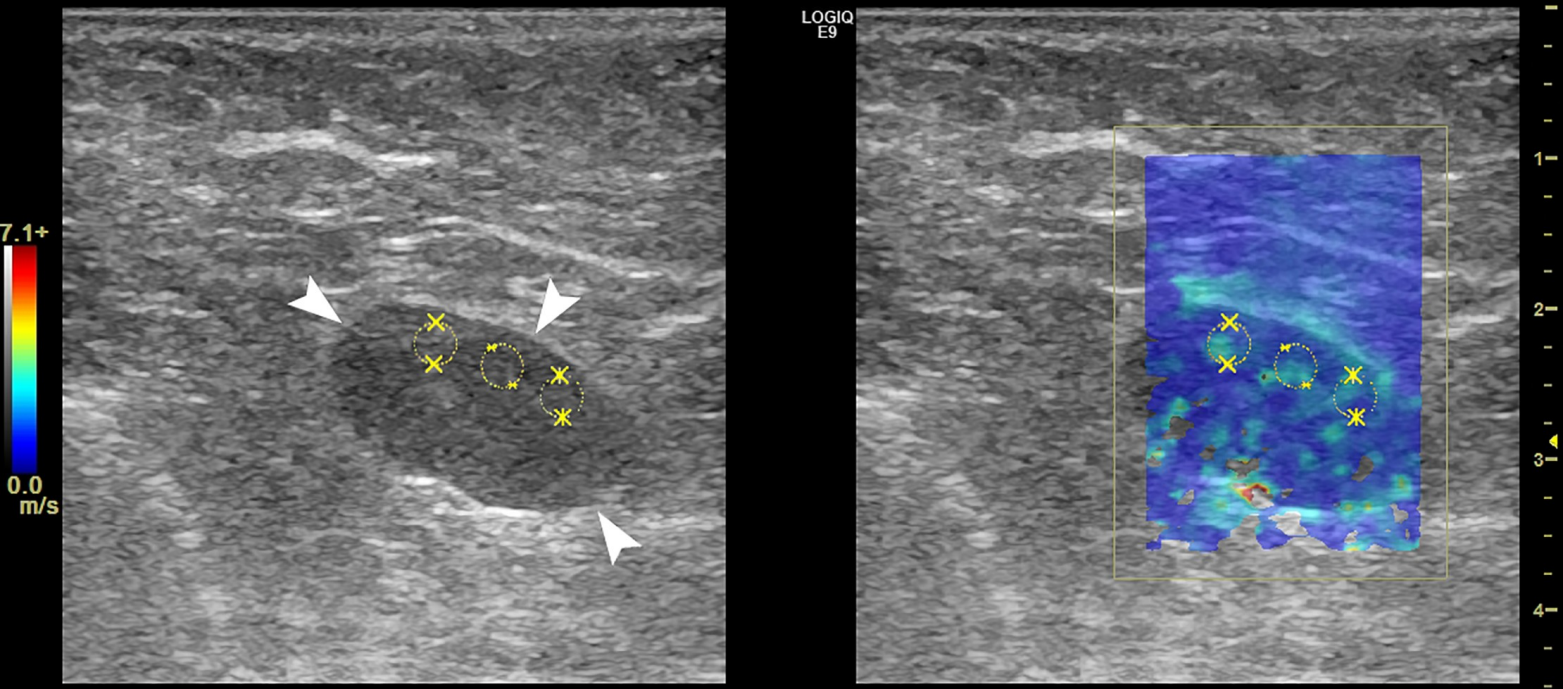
Left axillary B-mode (left) and shear wave speed map (right) images in a 60-year-old woman with a left breast mass and left axillary adenopathy identified on cardiac MRI. The US image shows an enlarged lymph node (white arrows) with a cortical thickness of 10.6-mm and absent hilum. The elasticity results calculated from the average of the 3 ROIs (yellow) were E_mean_ = 6.6-kPa and E_max_ = 20.1-kPa. FNA biopsy results showed a mixed lymphoid population consistent with a reactive intraparenchymal lymph node. Core needle biopsy of the breast mass resulted in a diagnosis of fibroadenoma with usual ductal hyperplasia.
